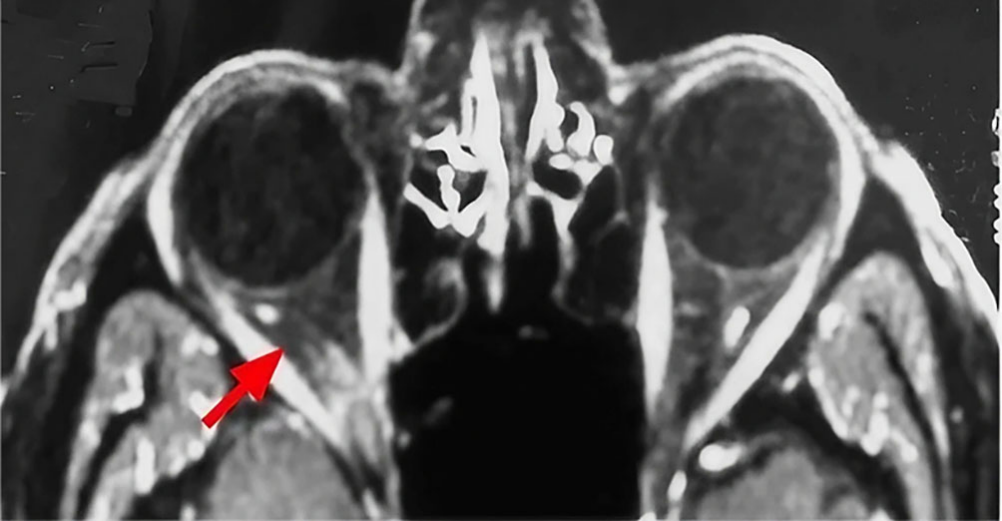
Postoperative axial MRI image demonstrating complete tumor resolution (red arrow).
